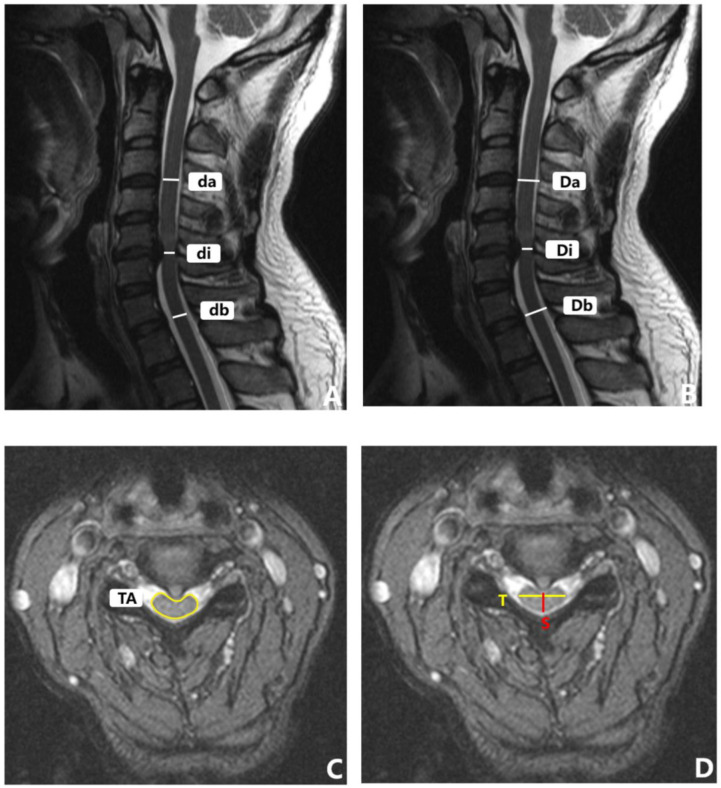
MRI parameter measurement of cervical spine. (A) MSCC. (B) MCC. (C) TA. (D) CR.
